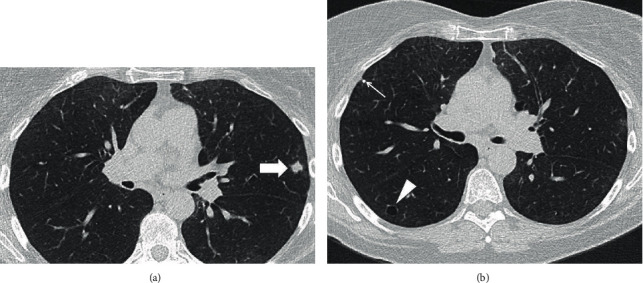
(a, b) Axial chest CT images show an irregular shape, 1.1 cm, left upper lobe nodule with spiculated margins (block arrow), and a thin-walled parenchymal cyst in right lower lobe (arrowhead). Please note mild centrilobular, paraseptal emphysema, and a tiny, calcified right upper lobe nodule (thin arrow).
